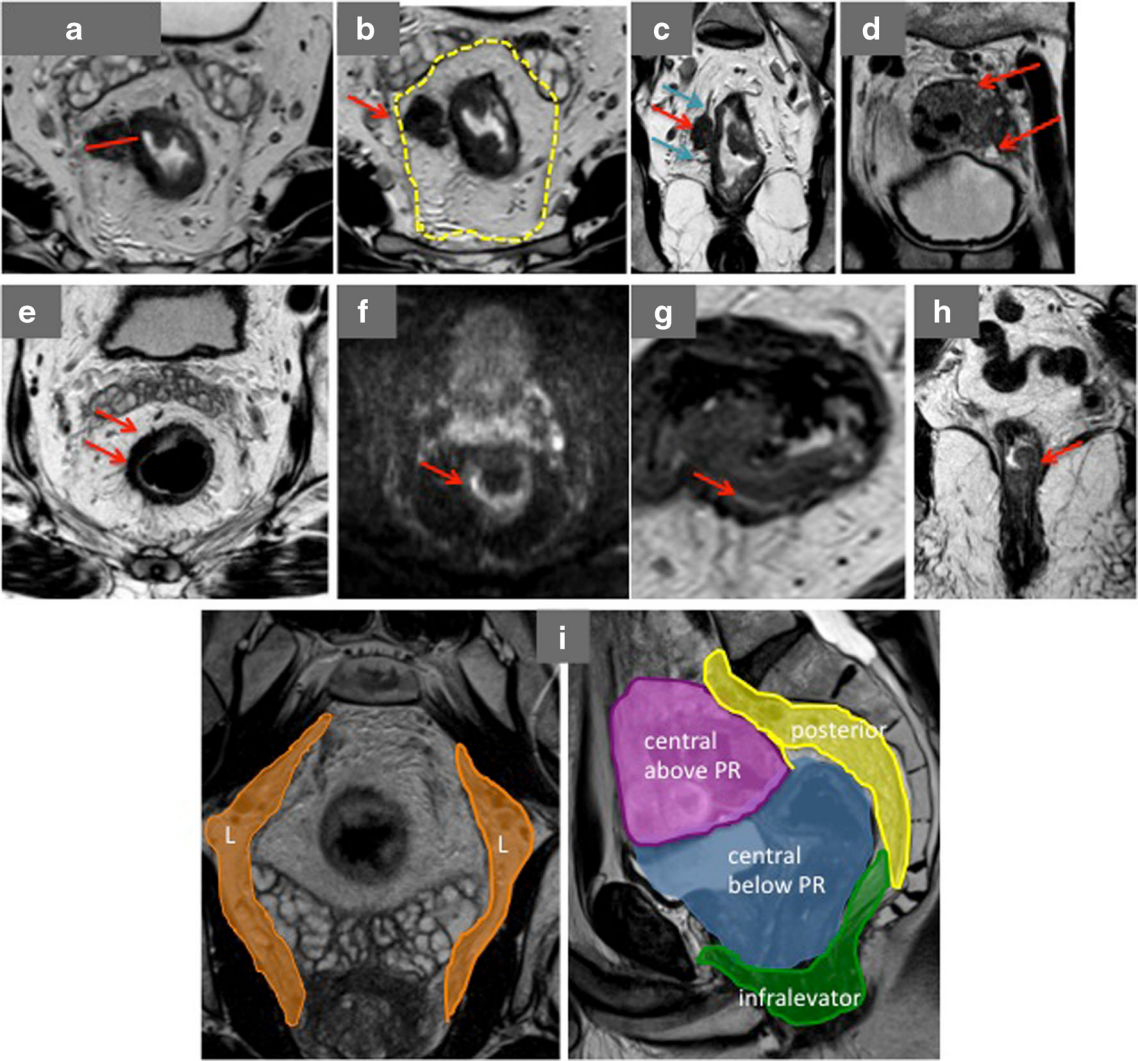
a An axial T2 image shows a semiannular tumour (before CRT) infiltrating rectal wall at 5–11 o’clock position. The extramural spread beyond the muscularis propria should be measured where it is the most advanced at 9–10 o’clock (*red line*). b A distance of less than 1 mm to the mesorectal fascia (*yellow line*) is considered as mrCRM + ve (*red arrow*). c A nodular deposit (*red arrow*) discontiguous with the rectal wall is located along the extramural veins (*blue arrow*). The extramural veins are also expanded and have intermediate signal within them—features of EMVI. d High signal intensity areas (*red arrows*) within the tumour are suggestive of mucinous content. e Low-density fibrosis is noted at the level of the treated tumour (*red arrows*) after CRT (the same patient as at Fig. 1a). f The same level of tumour as in Fig. 1e. High *b* value (1000) DW image shows evidence of restricted diffusion (*red arrow*); despite these findings, the patient has been enrolled in the deferral of surgery trial and is disease free for 3 years. g High-resolution axial MRI shows a sessile lesion infiltrating rectal wall at 4–6 o’clock position. A high signal intensity line (*red arrow*) is visible between the tumour and muscularis propria, which represent partially preserved submucosal layer. h A low rectal tumour confined to the part thickness of muscularis propria, indicating that the intersphincteric or mesorectal planes are safe. i Pelvic compartments are marked on these two high-resolution MR images (*L*—left compartment, central above the peritoneal reflection (*PR*), central below the *PR*, posterior, infralevator)
